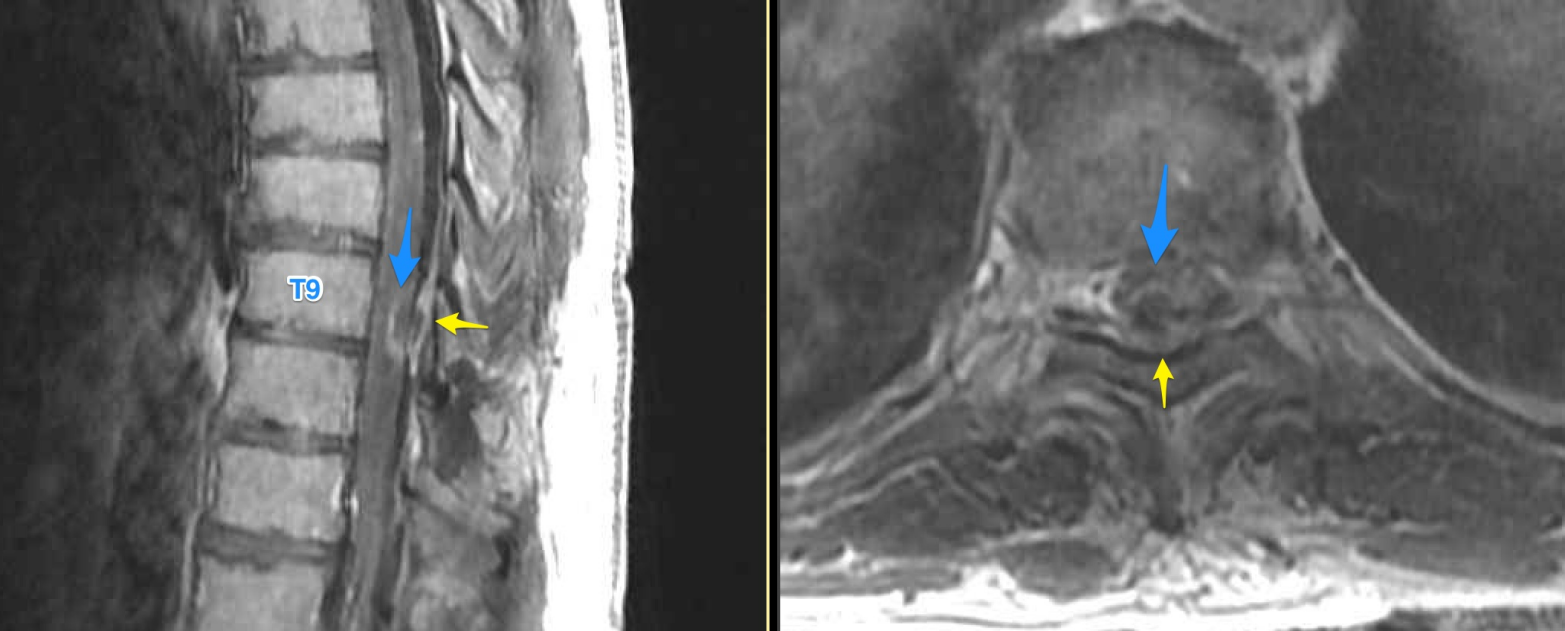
T1 post-contrast sagittal and axial thoracic MRI showing an intramedullary enhancing lesion (blue). Epidural fluid collection (yellow) status post stimulator lead removal is also shown. MRI: magnetic resonance imaging
